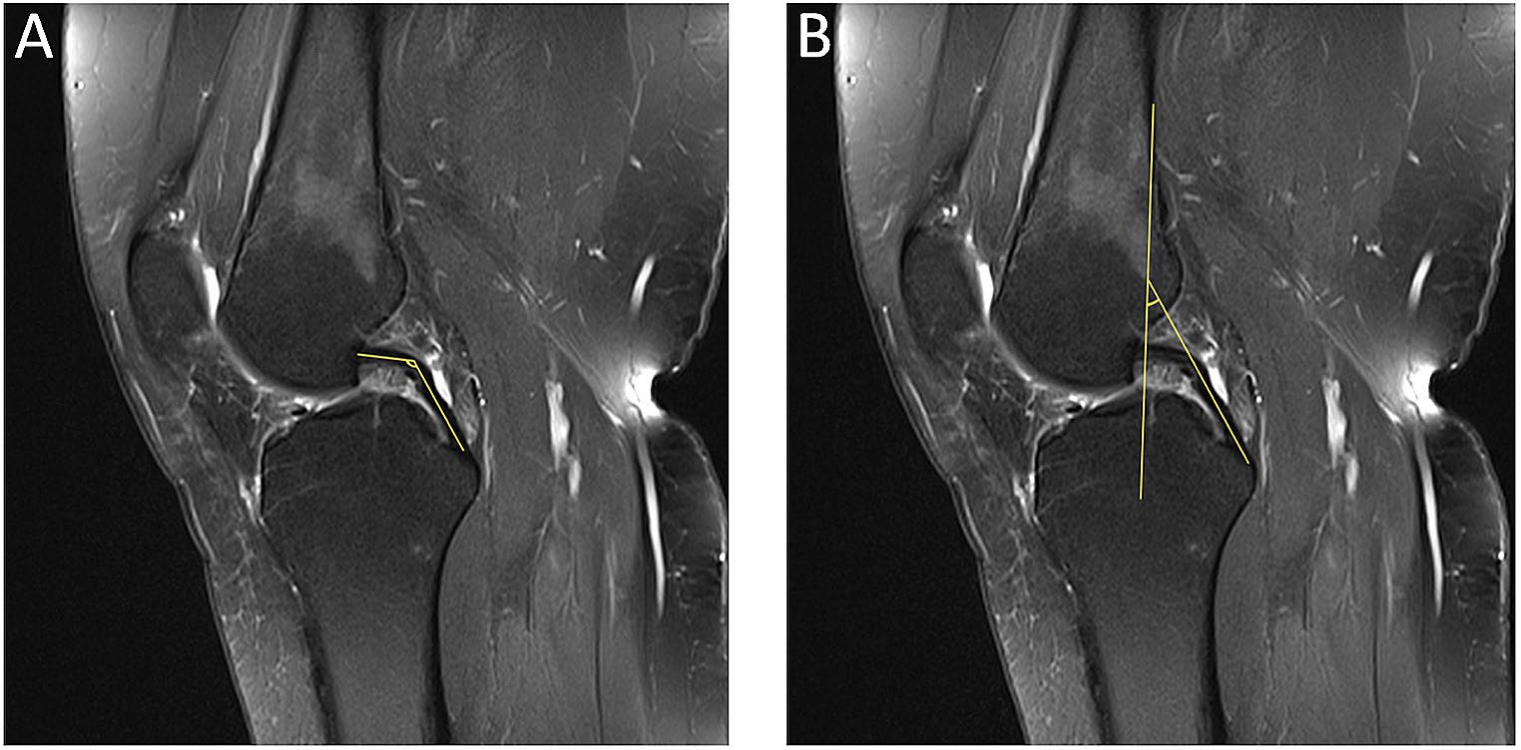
Sagittal T2-weighted MRI of the knee showing PCLA (a) and PCL-PCA (b) views
